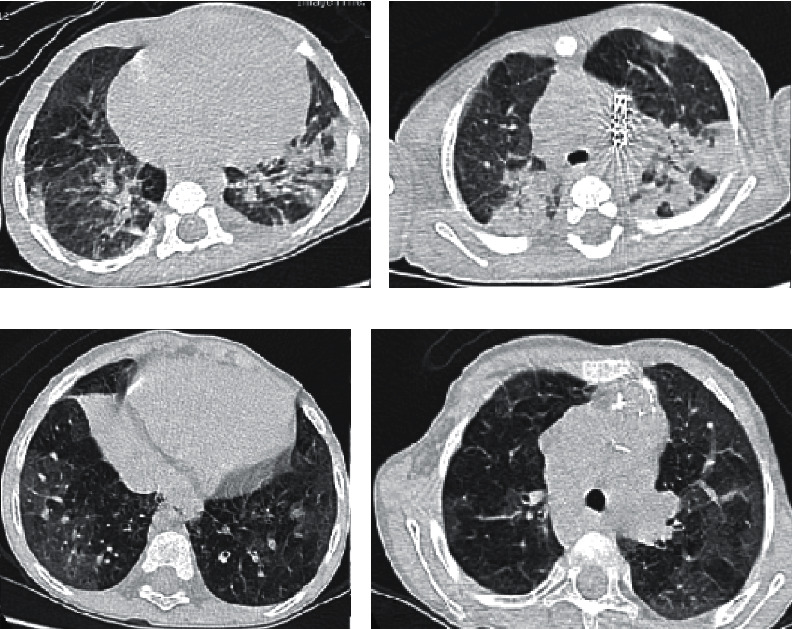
CT scan (a, b) of patient 1 with subpleural alveolar consolidations, deformed interlobular septal thickening, and mosaic attenuation. Ductal stent can be seen in (b). CT scan (c, d) of patient 2 with diffuse ground-glass opacities.
